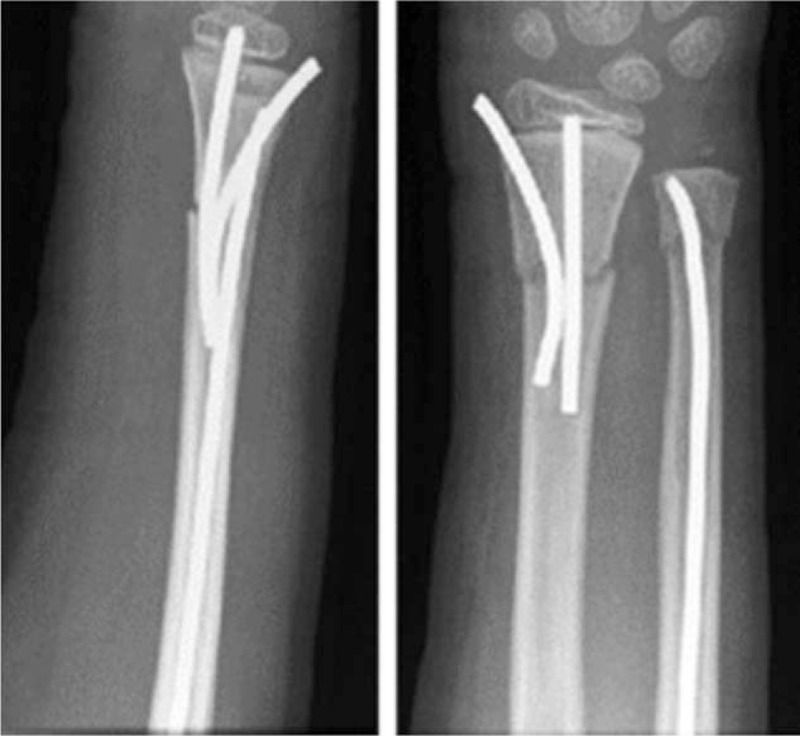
Lateral and anteroposterior radiographs show the SESIN method. SESIN = short titanium elastic stable intramedullary nailing.
